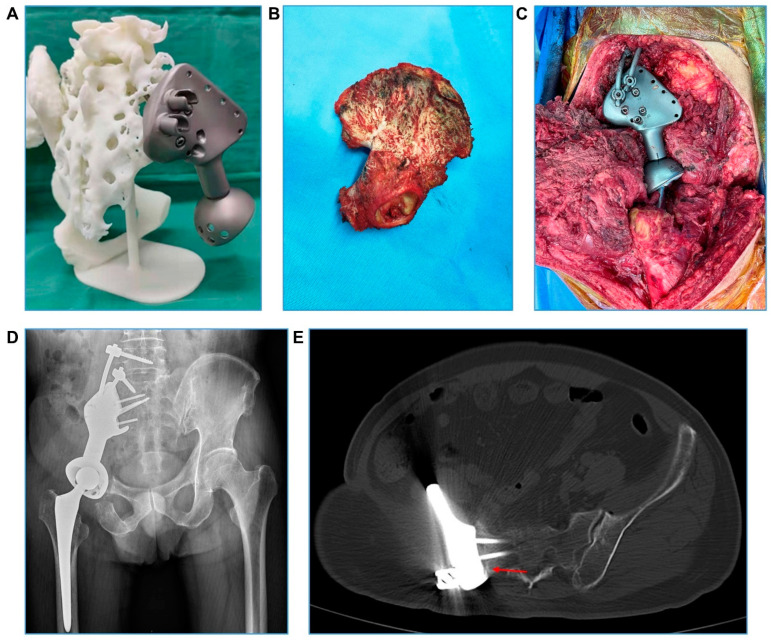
Case 2. Intra- and post-operative images. (A) Finished 3D-printed hemipelvic endoprosthesis. (B) The total en bloc removed ilium and acetabulum. (C) The 3D-printed prosthesis is installed and fixed. (D) X-ray shows a good implantation effect at the visit 6 months post operation. (E) CT shows good bone ingrowth and fusion at the prosthesis and bone interface (red arrow) at the visit 10 months post operation.
